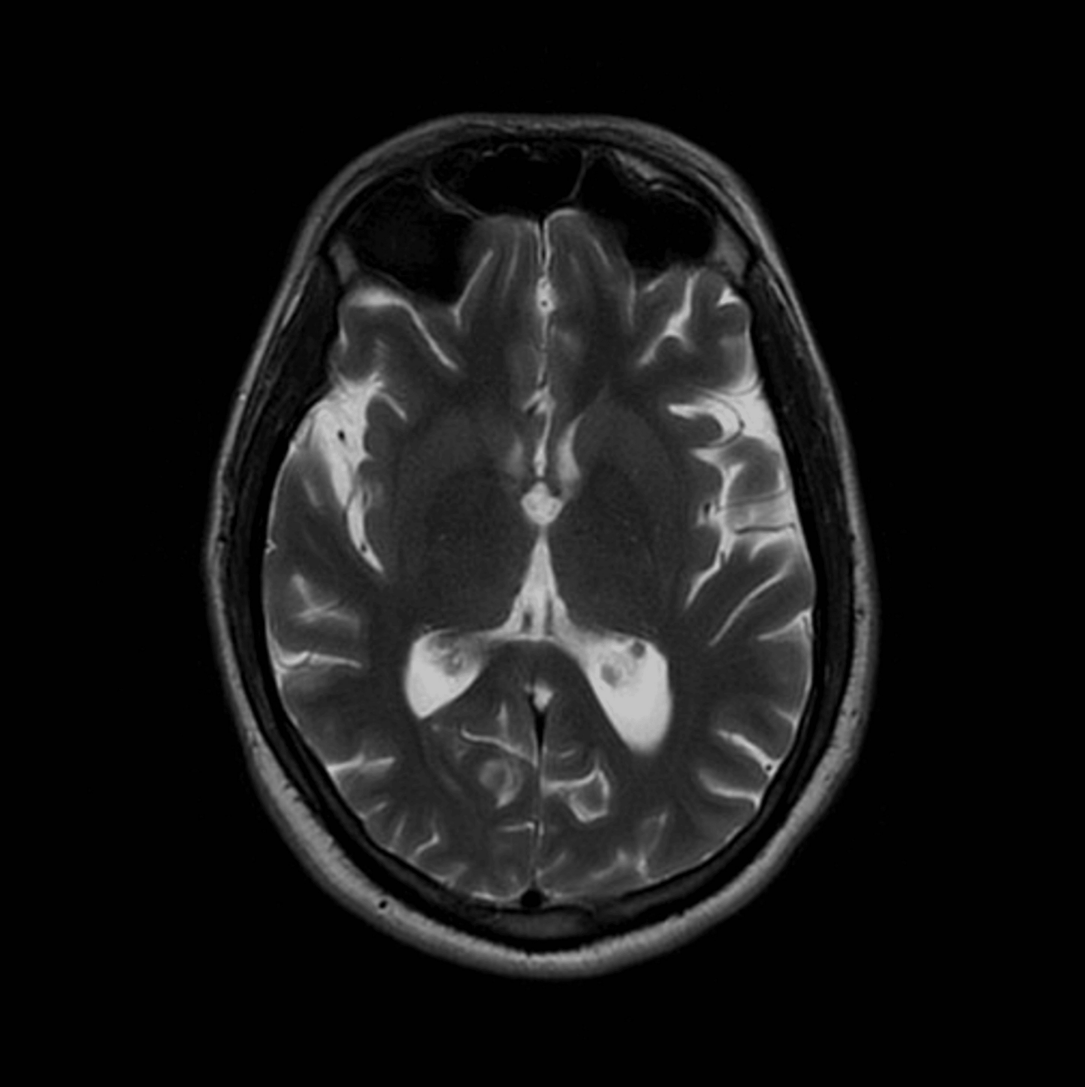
A magnetic resonance imaging scan with contrast of head Showing normal scan, no intracranial pathology was identified.
